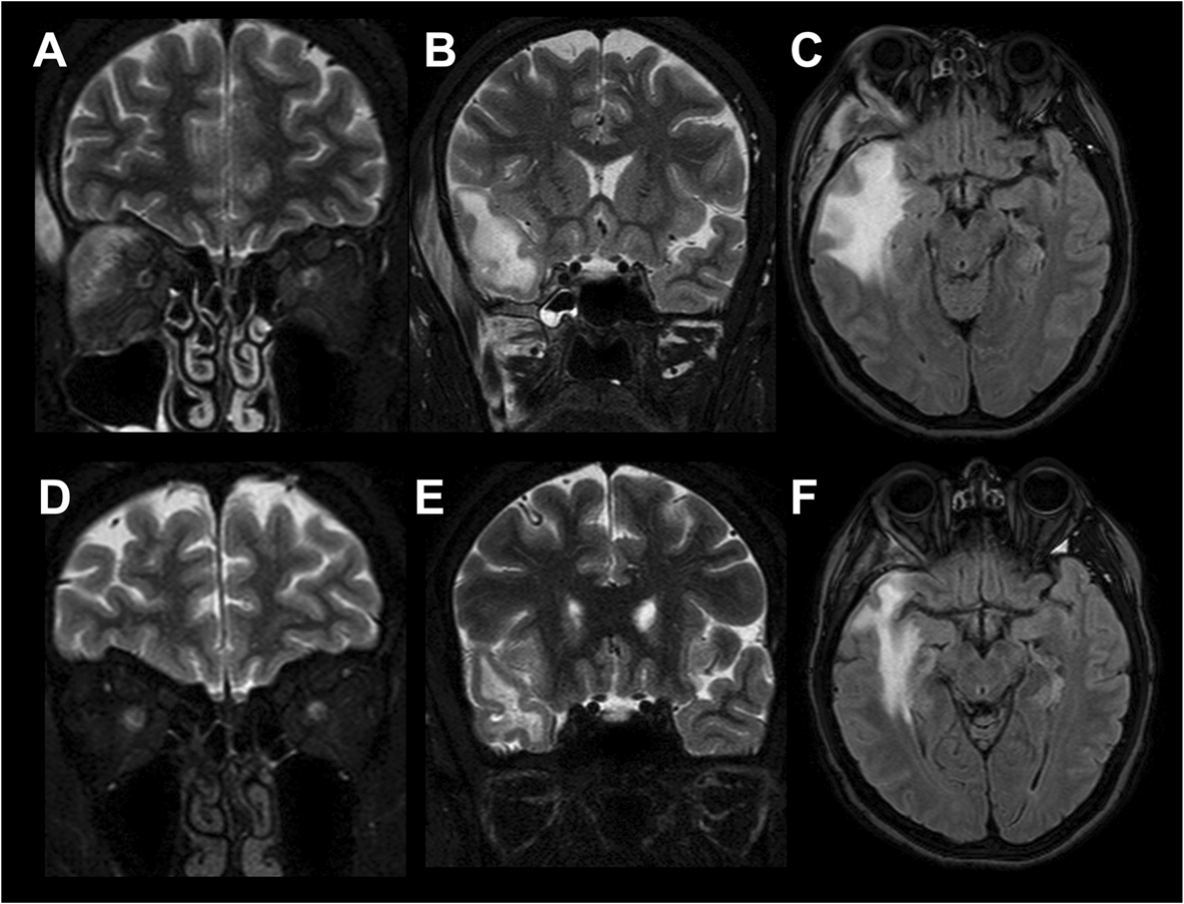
Orbital and extraorbital involvement before and after steroid therapy. Upper row: coronal STIR (a, b) and axial FLAIR (c) MR images before treatment. Lower row: coronal STIR (d, e) and axial FLAIR (F) MR follow-up images after steroid therapy. Evidence of marked enlargement and edema involving the lateral rectus muscle in the right orbit, with infiltration of the contiguous fat (a), and the temporal and pterygoid muscles in the ipsilateral masticator space (b), all showing complete regression at follow-up (d, e). The massive vasogenic edema of the anterior portion of the temporal lobe evident in the initial MR scan (c), also shows dramatic reduction at follow-up (f)
